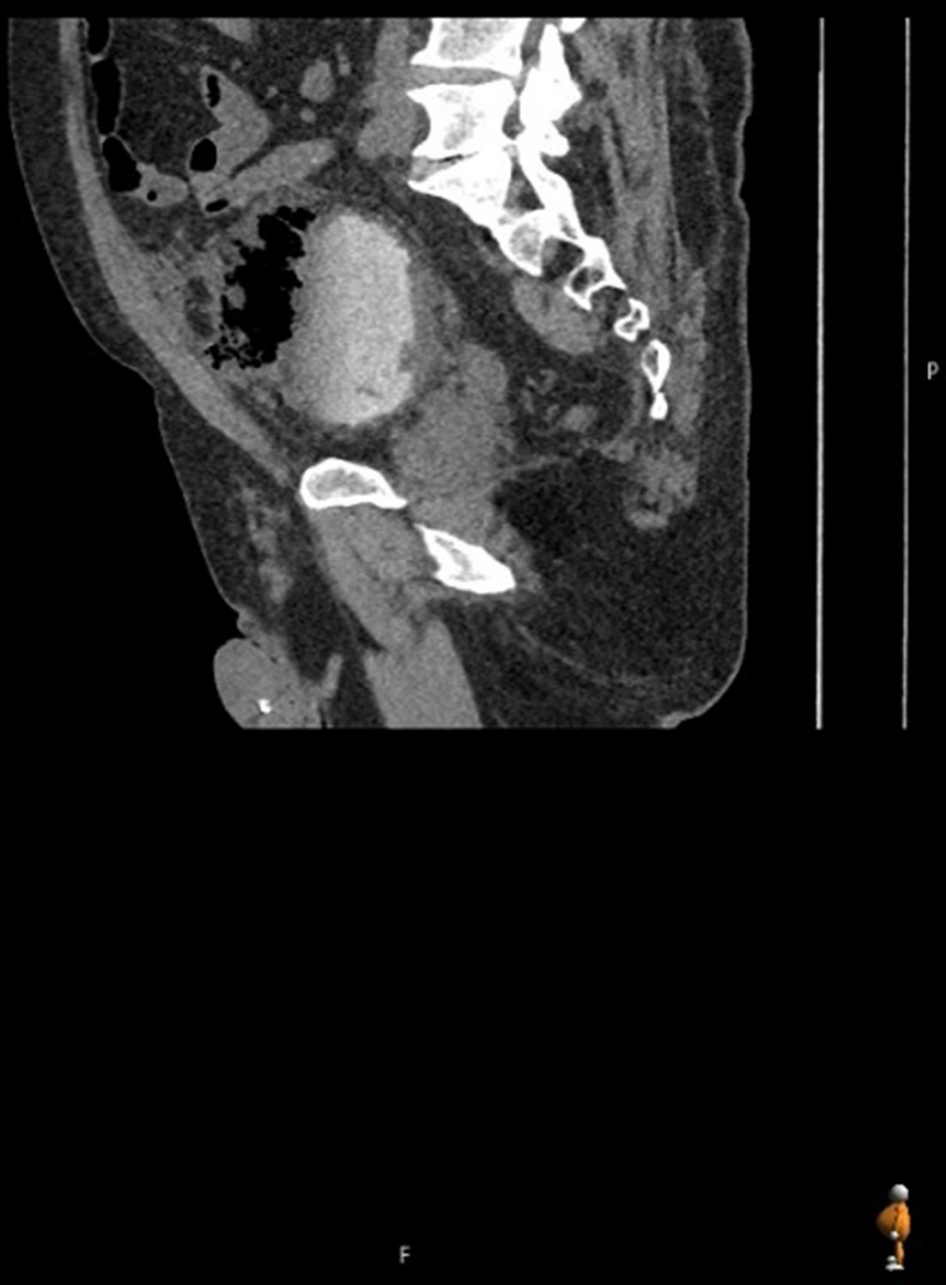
Second sagittal delayed phase CT (Day 8 of admission), on soft tissue window
